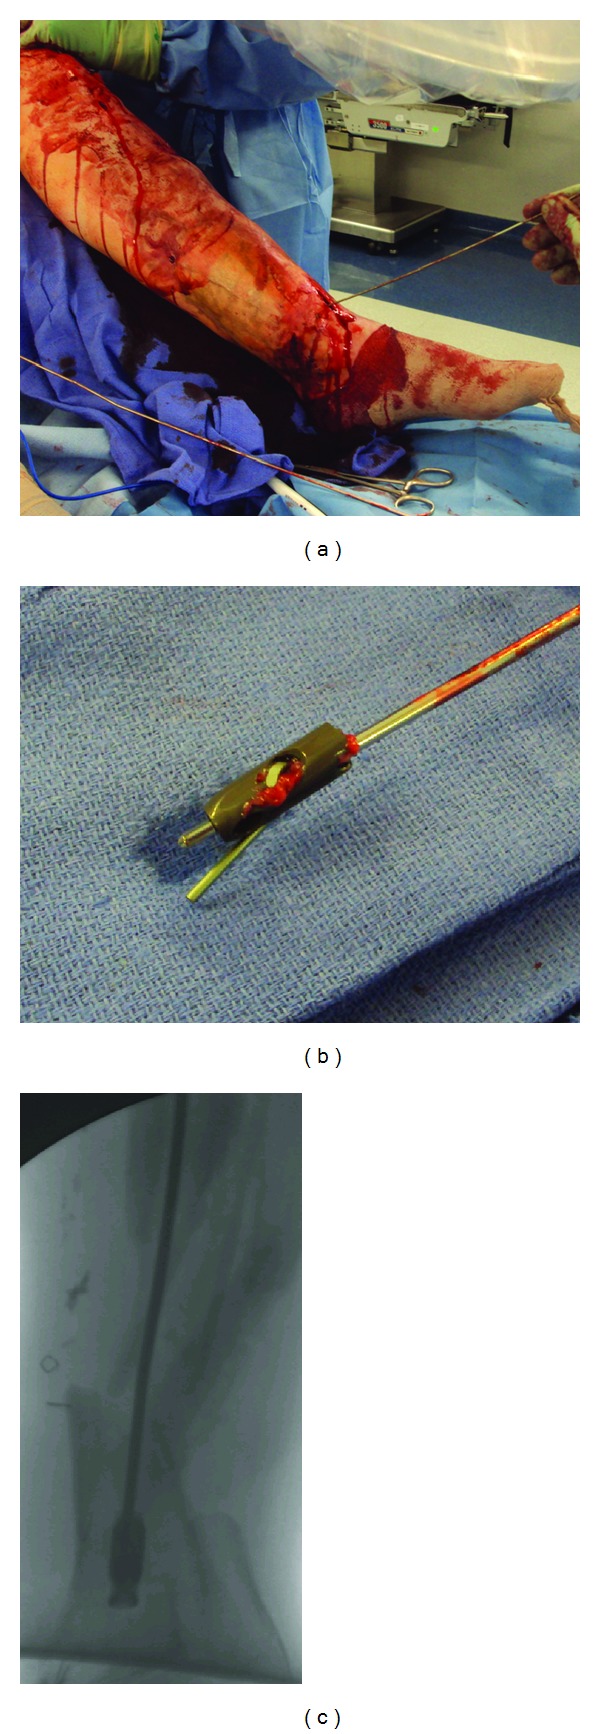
(a) Insertion of the flexible nail from the locking hole. The flexible nail was then cut at a distance of about 2 inches. (b) The broken piece was removed with the hook and the piece of the flexible nail wrapped around the hole of the locking screw. (c) Radiograph during extraction of the broken piece.
